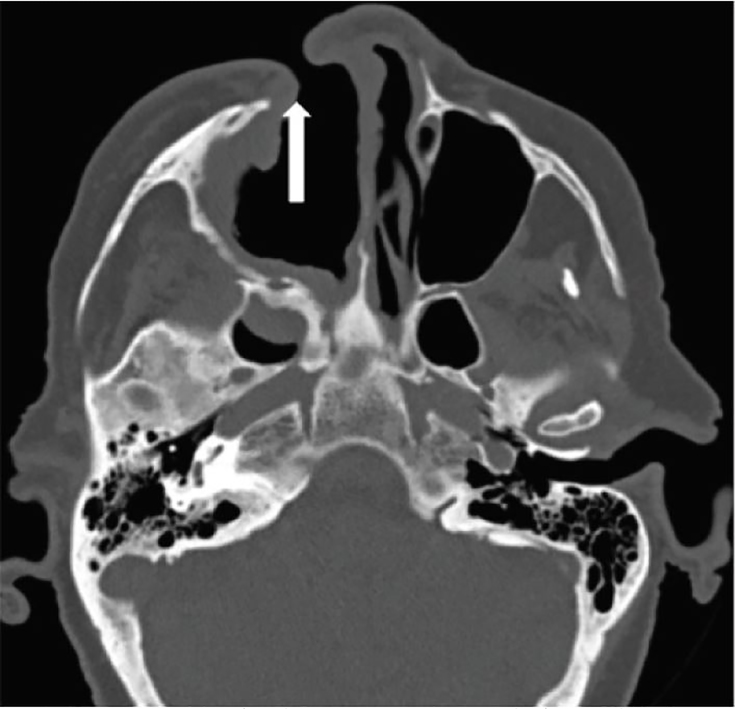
Axial computed tomography demonstrating anterior maxillary wall sinus erosion (arrow).
